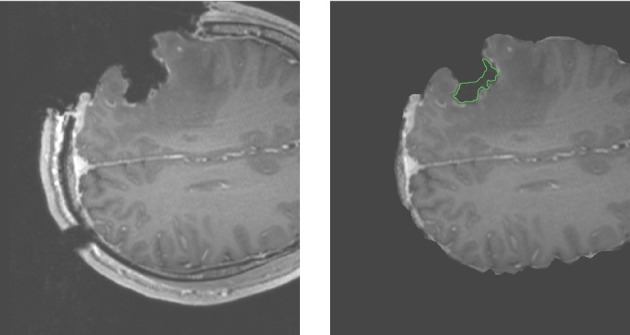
Enhanced views of the intra-op MRI (case 13). The left figure demonstrates the brain prior to the extraction from the skull with background noise clearly visible. The right figure shows the brain following extraction from the skull. The background noise is mostly removed except the green demarcated region near the edges of the tumor cavity.
